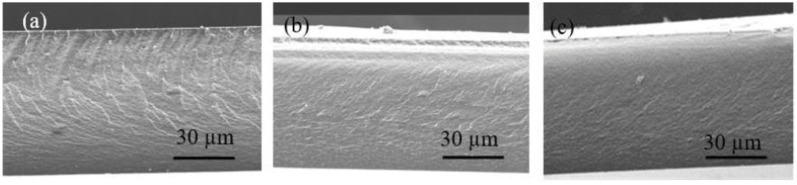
Cross-section SEM images of dry PIMs with a composition of 60%IL–40%CTA. (a) AlqCl; (b) AlqNO_3_; and (c) AlqSCN.
